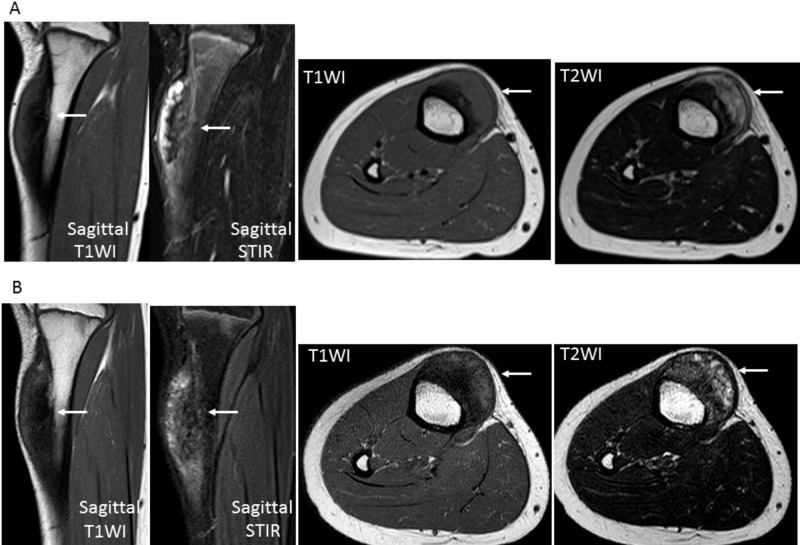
Magnetic resonance imaging (MRI) of the tibial periosteal osteosarcoma before and after neoadjuvant chemotherapy (NAC) A) Pre-NAC MRI shows the mass as a hypointense inner part and isointense outer part on axial and sagittal T1-weighted images and hypointense inner part and hyperintense outer part on axial T2-weighted images and sagittal STIR; B) Post-NAC MRI shows the mass as a growing hypointense inner part and isointense outer part on axial and sagittal T1-weighted images and growing a mixed hypo- and slight hyperintense inner part and hyperintense outer part on axial T2-weighted images and sagittal STIR. STIR: short T1 inversion recovery; T1WI: T1-weighted images; T2WI: T2-weighted images
